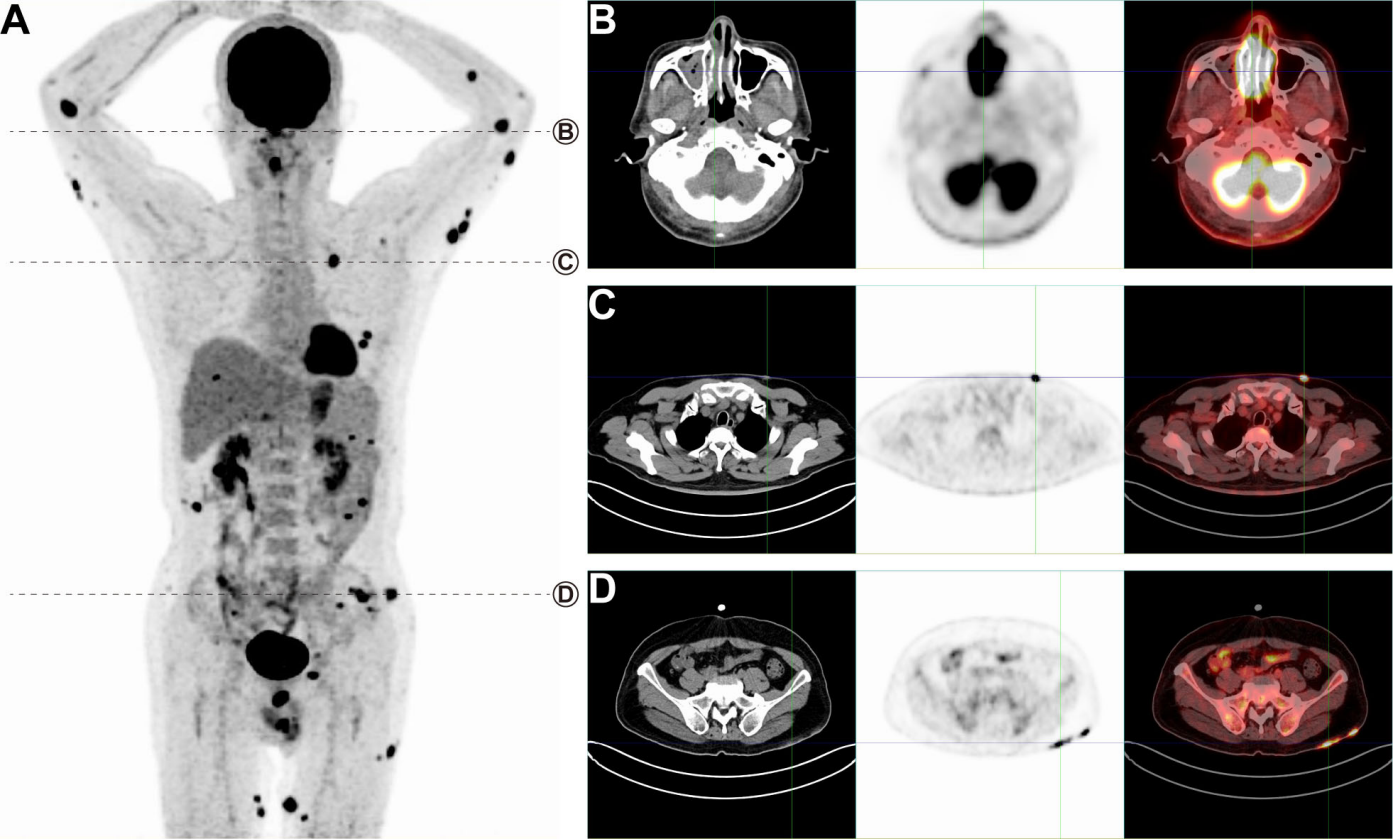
PET/CT imaging before treatment demonstrating extensive FDG uptake in multiple regions, indicating active disease involvement. (A) A maximum intensity projection (MIP) whole-body image shows widespread hypermetabolic lesions. (B) Significant ^18^F-FDG uptake in the nasal cavity (SUVmax 27.6) suggests aggressive disease in the nasal region. (C) Lesions on the left chest wall (SUVmax 24.1) and (D) left buttock (SUVmax 15.7) also demonstrate intense FDG uptake, indicative of extranasal disease spread.
